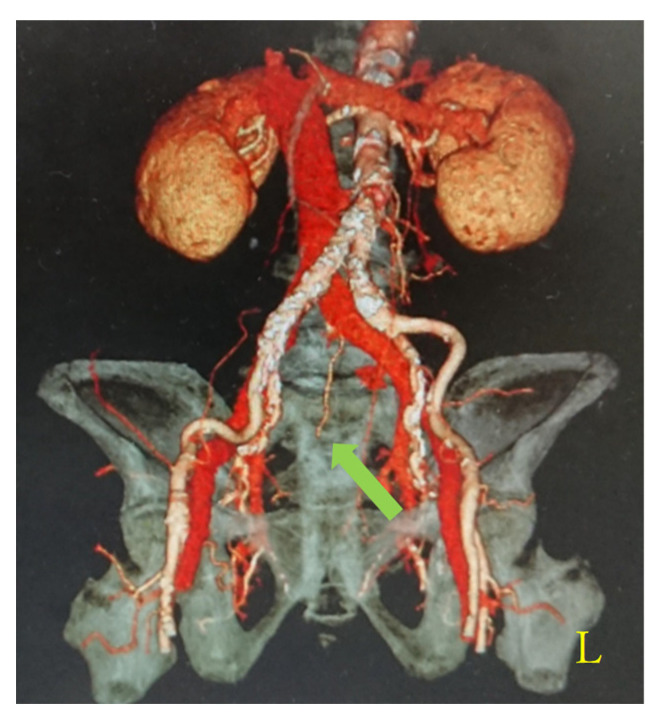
Representative contrast-enhanced CT image demonstrating the presence of a median sacral vein. Arrows indicate the median sacral vein. This image represents a typical case observed in this study.
